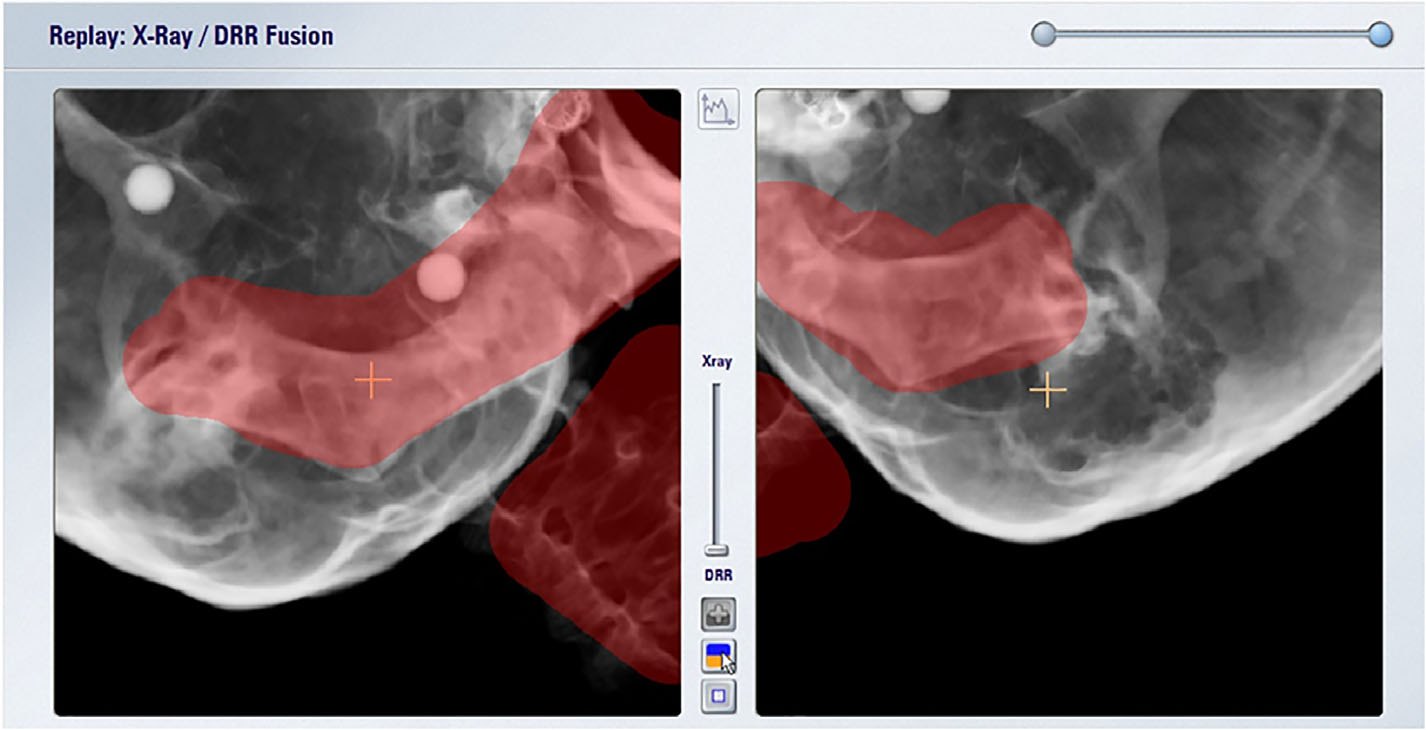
Masking of the mandible and cervical vertebrae within the scanning area of the ExacTrac X‐ray system. The masking area is red Abbreviation: DRR, digitally reconstructed radiograph.
